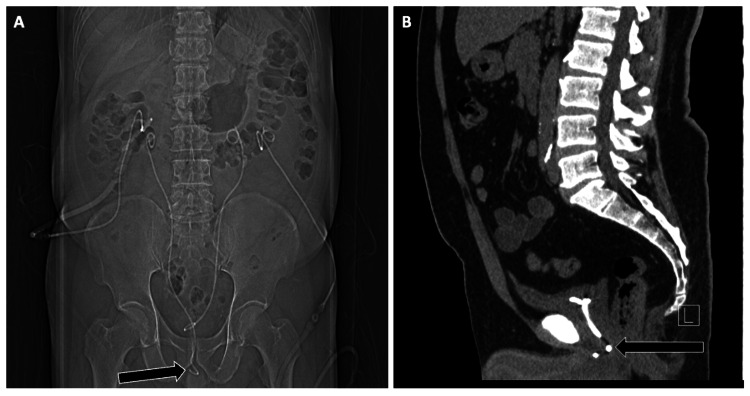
Distal Migration of a Ureteral Stent Into the Prostatic Urethra Imaging of a malpositioned right ureteral stent demonstrating distal migration. (A) Supine abdominal radiograph shows the stent tip projecting below the pubic symphysis (arrow). (B) Sagittal non-contrast CT confirms distal stent migration with the pigtail loop extending into the prostatic urethra (arrow). Recognition of the abnormal distal course is critical, as urethral migration can cause pain, hematuria, and urinary obstruction.
